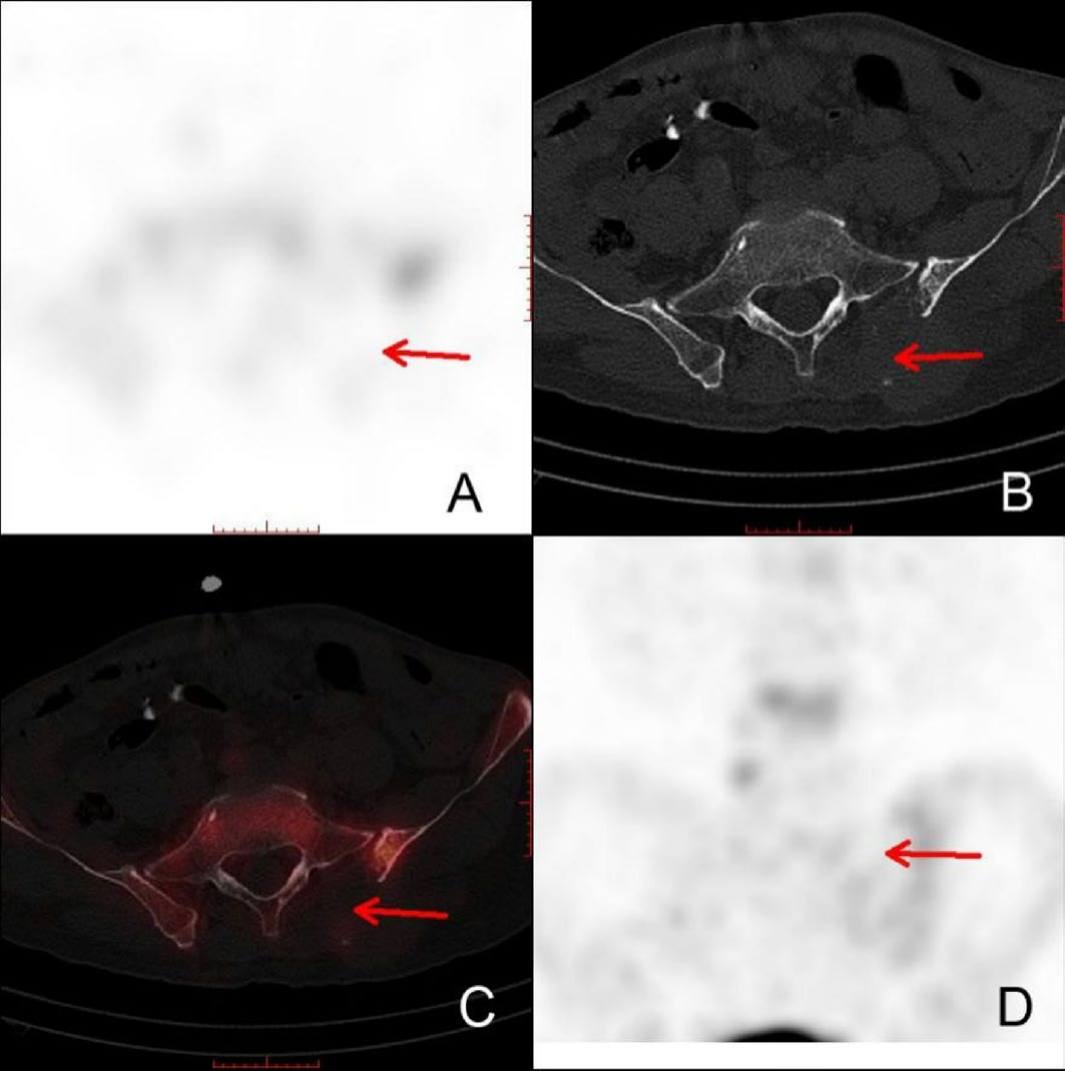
Presence of RCZLs on bone SPECT/CT scan.
